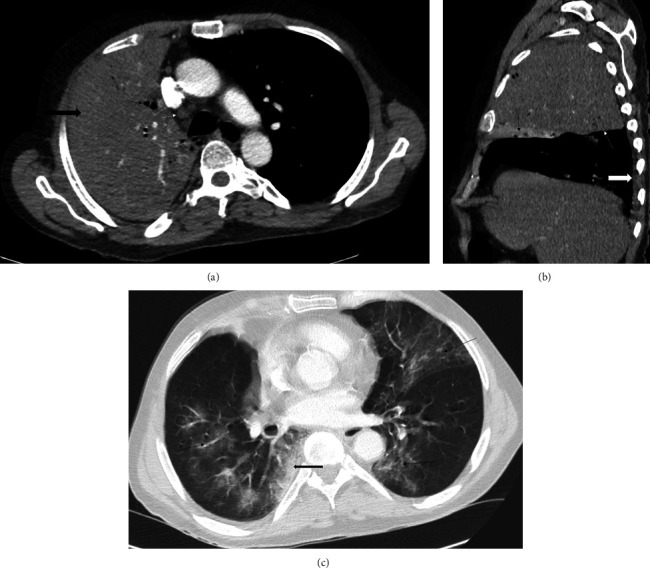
(a) Axial contrast enhanced CT image with mediastinal window settings demonstrating consolidated RUL (thick black arrow) with foci of cavitation, one of which is indicated with the thin black arrow. Mildly enlarged pretracheal-retrocaval lymph node (thin white arrow). (b) Contrast-enhanced CT reconstruction through the right hemithorax with mediastinal window settings. Expanded, consolidated RUL with depression of the oblique fissure indicated by a thin white arrow. Collapsed RML demonstrating enhancement, contrasting with the hypo-enhancing consolidated RUL (thin black arrow). Small right pleural effusion (thick white arrow). (c) Axial CT image with lung window settings demonstrating bilateral multilobar foci of ground-glass density (thin black arrows) and a focus of RLL paravertebral subpleural consolidation (thick black arrow).
